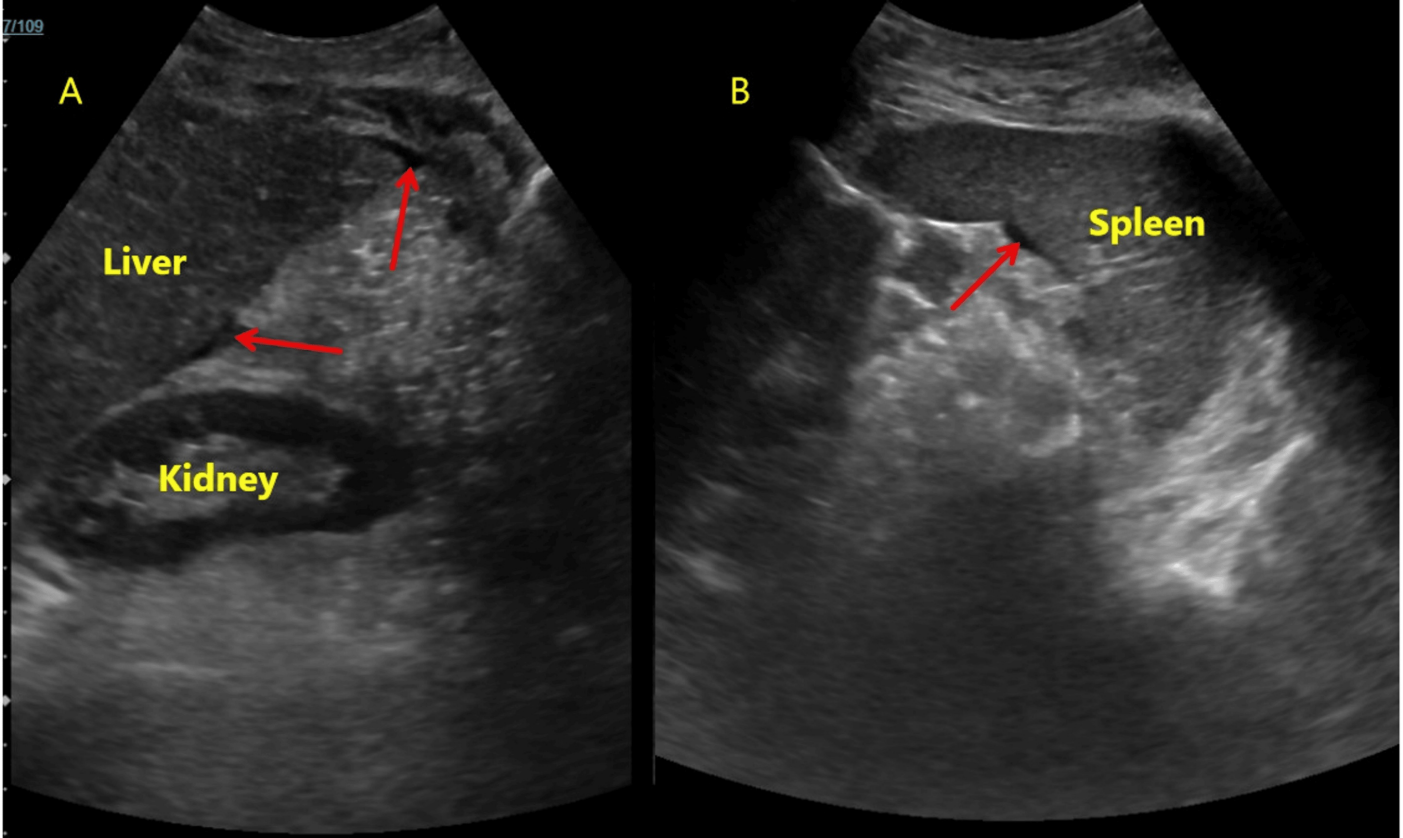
Ultrasound images Minimal fluid was demonstrated in the right (A) and left (B) upper quadrant sonographic images, with no evidence of cirrhosis (red arrows).
